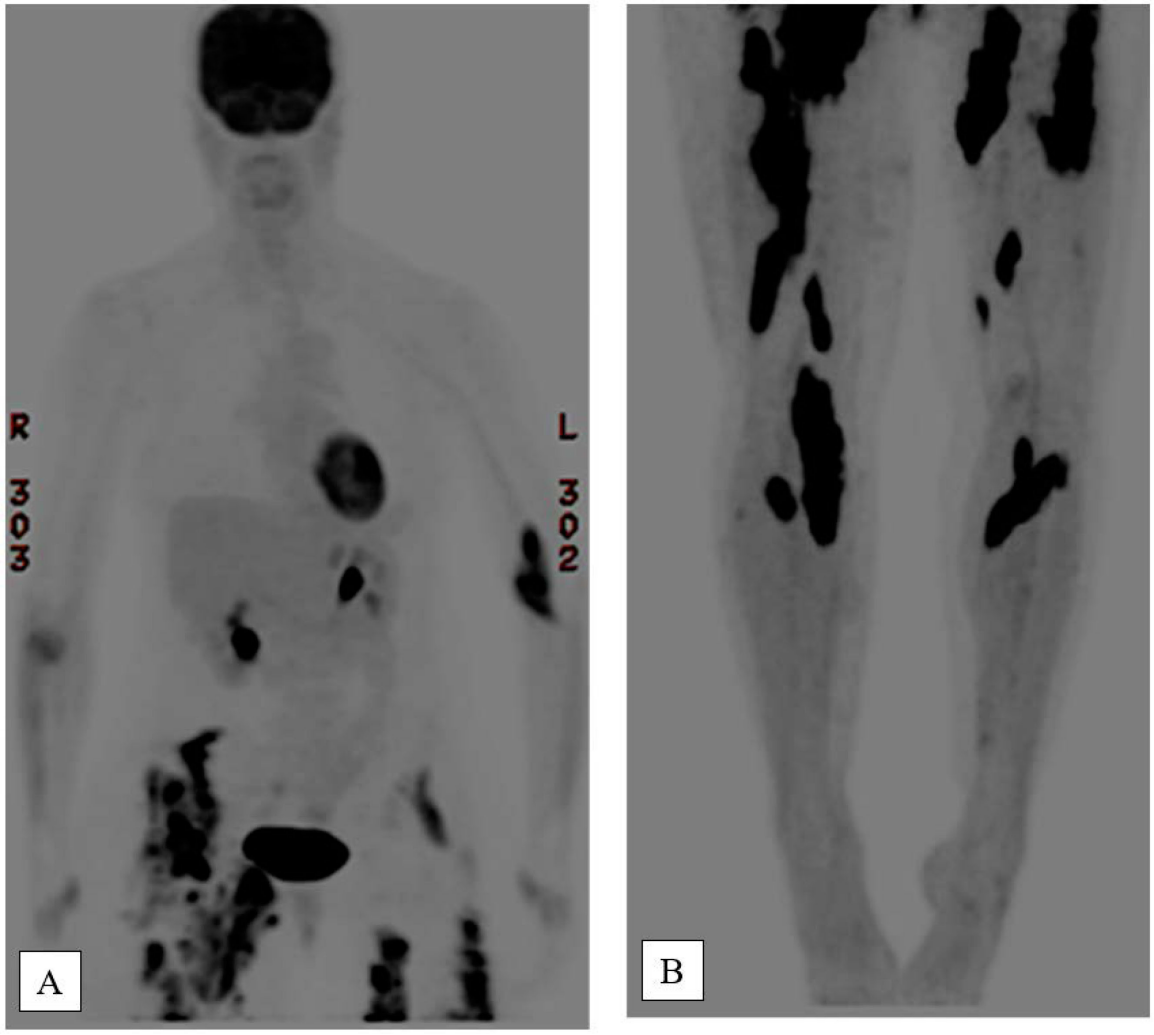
Positron emission tomography/computed tomography images of upper (A) and lower (B) body taken in late 2014, showing increased tracer uptake in the proximal right radius with maximum standardized uptake value (SUV) of 5.5; linear increased uptake in the right supinator (SUV of 3.1); left antecubital fossa (SUV of 12.0); increased tracer activity in the right lower abdomen/pelvis (SUV of 17.1); right thigh adductors (SUV of 14.1); right popliteal region (SUV of 14.4); left hip with increased uptake (SUV of 8.6); left adductors (SUV of 15.3); and left quadriceps (SUV of 13).
